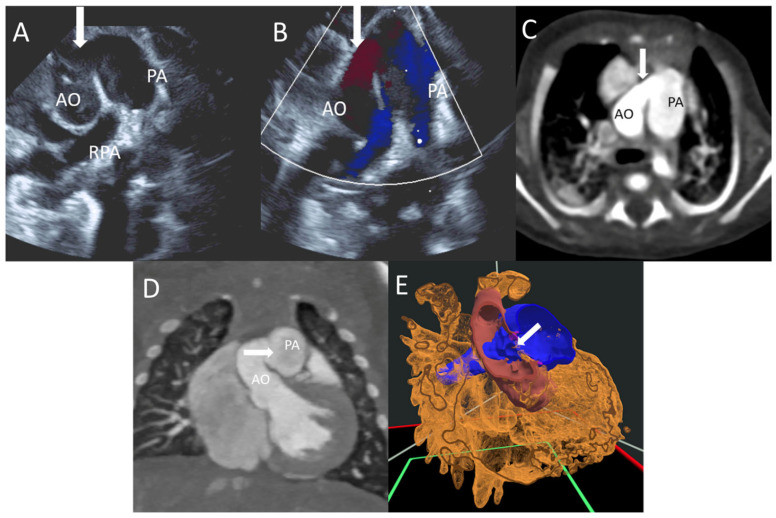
(A,B) TTE 2D and color of the AP window arrow; (C,D) CT imaging of the AP window; (E) VR segmentation with the arrow pointing the AO and PA connection.
